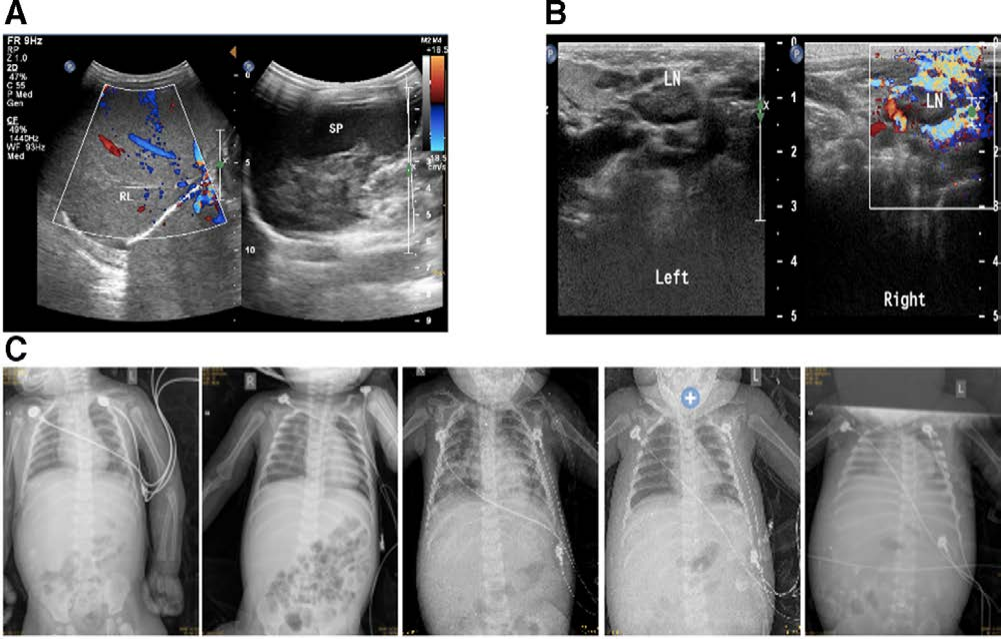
Imageological examinations of the proband with the progression of disease. (A) Results of abdominal color ultrasound. (B) Results of neck color ultrasound. (C) Results of chest X-ray. There were 5 times of examine results during hospitalization listed in chronological order from left to right.
